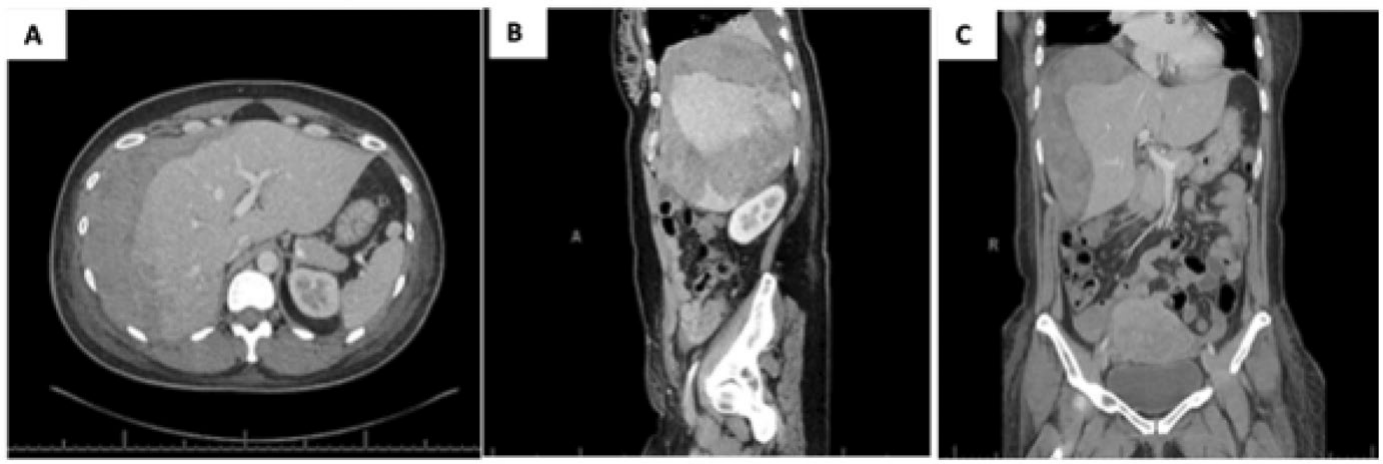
(A–C) Patient in Case 2. Abdominal CT scan on POD 5 demonstrated a large hepatic hematoma measuring 21 × 11 × 9 cm along with areas of hypoattenuation in segments 4–8, possibly representing areas of hepatic infarction. 2A. Transverse view. 2B. Sagittal view. 2C. Coronal view.
